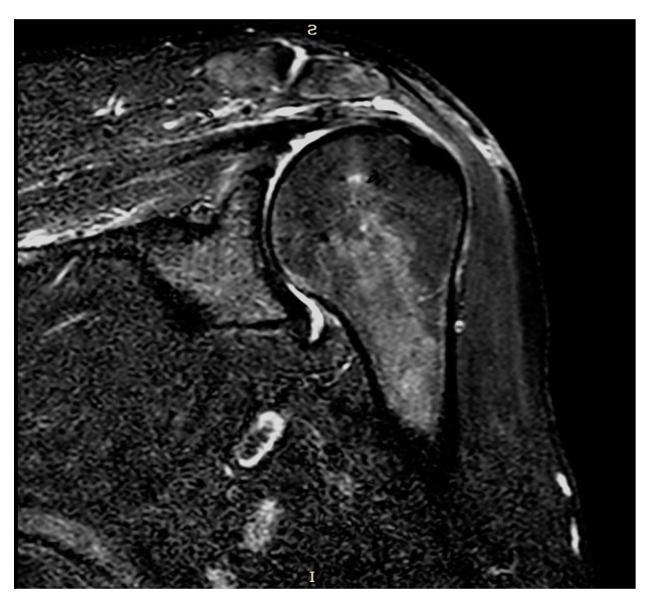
Coronal STIR-weighted MRI.
